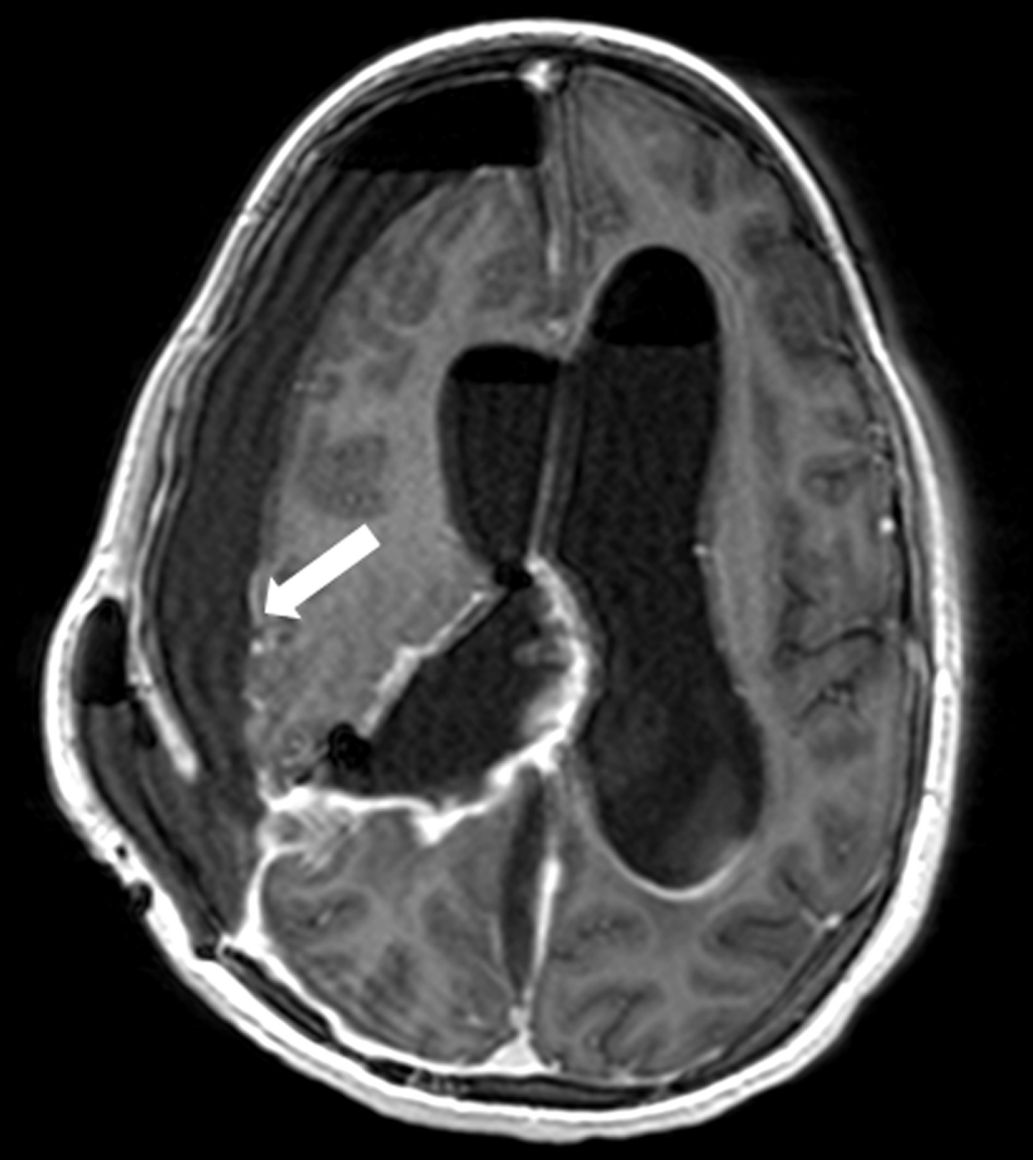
T1-weighted gadolinium-enhanced axial MRI Note the right subdural enhancing collection (arrow). MRI: magnetic resonance imaging
